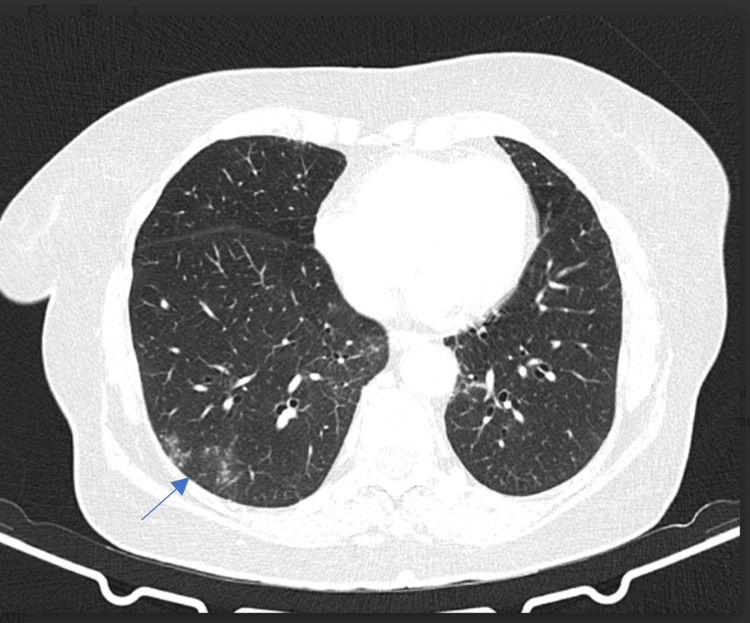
Immune-related pneumonitis. Lung CT-scan showing ground-glass opacities in the right lower lobe, consistent with immune-related pneumonitis grade 1 (CTCAE).
